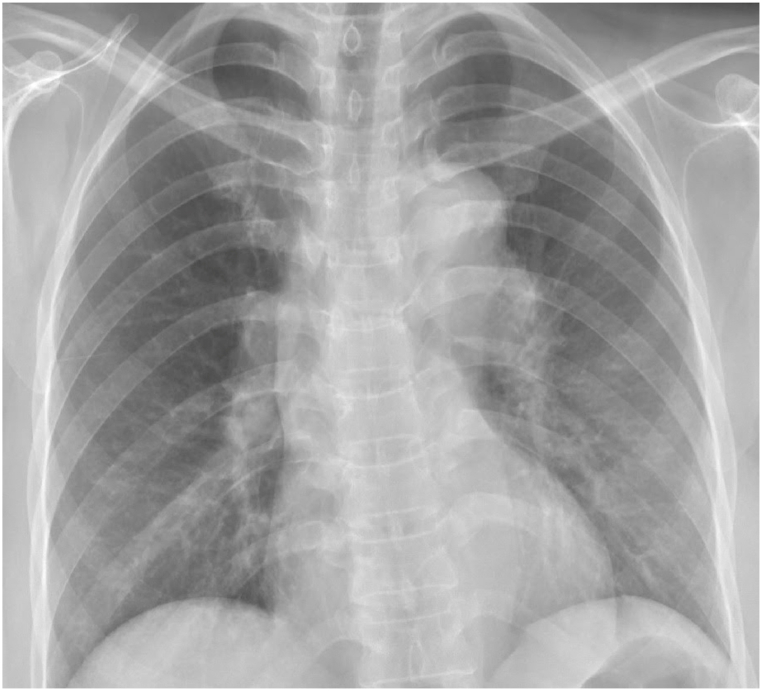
Chest radiograph demonstrating bilateral hilar adenopathy.
